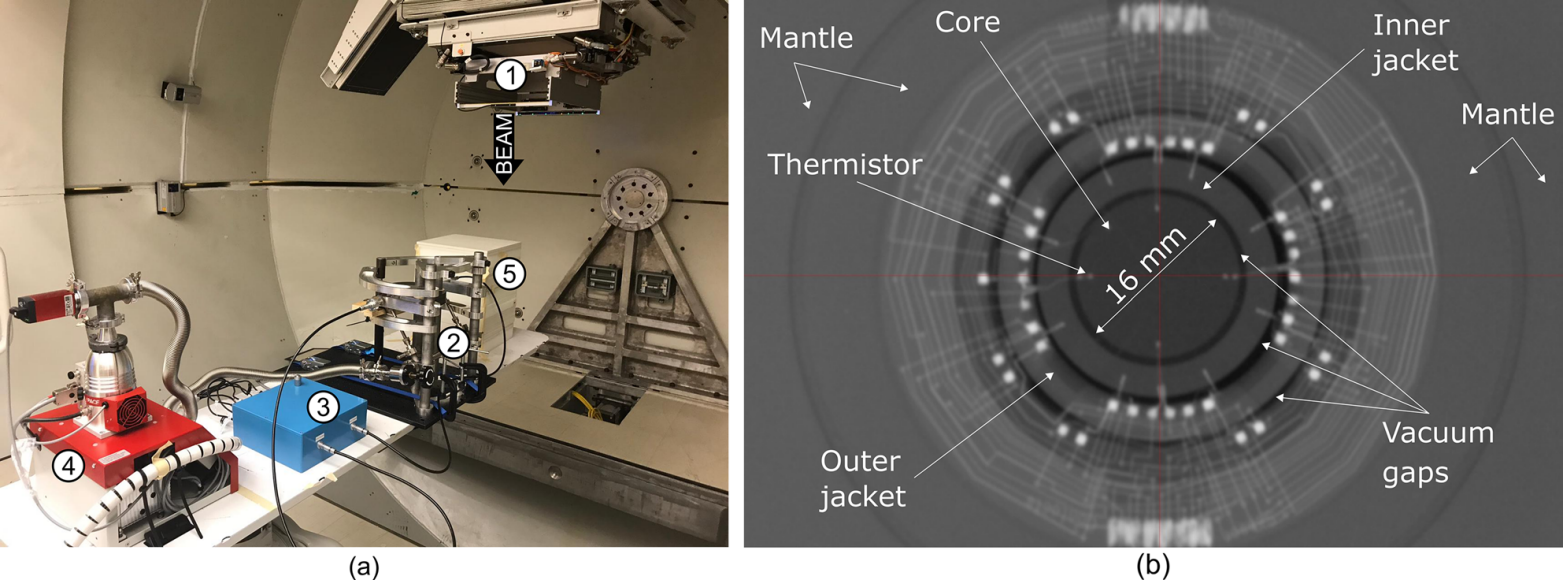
Experimental setup mounted on the patient couch and radiograph of the NPL PSPC. (a) (1) Gantry, (2) NPL PSPC, (3) measurement instrumentation for the NPL PSPC, (4) vacuum pump, (5) ionisation chamber setup. (b) Radiograph of the front view of the NPL PSPC^44^.
